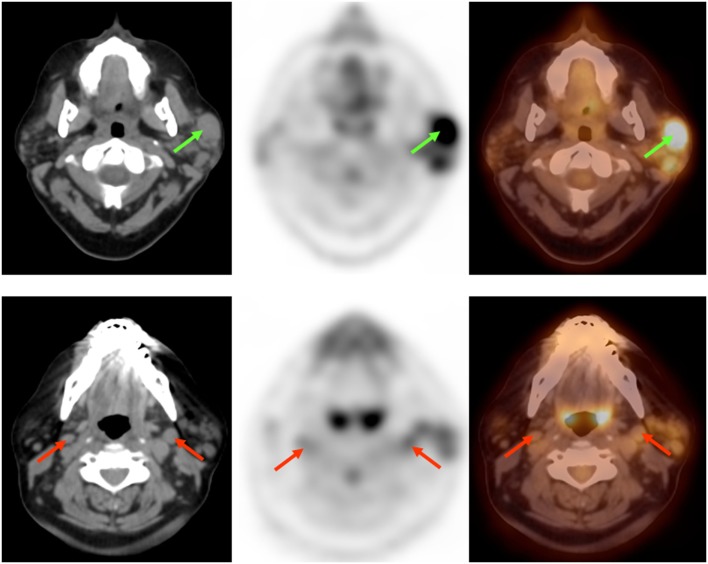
F-18 Fluorodeoxyglucose position emission tomography/computed tomography demonstrated FDG avid predominately left parotid nodule (green arrows) and cervical lymph nodes (red arrows), suspicious for lymphoma.
